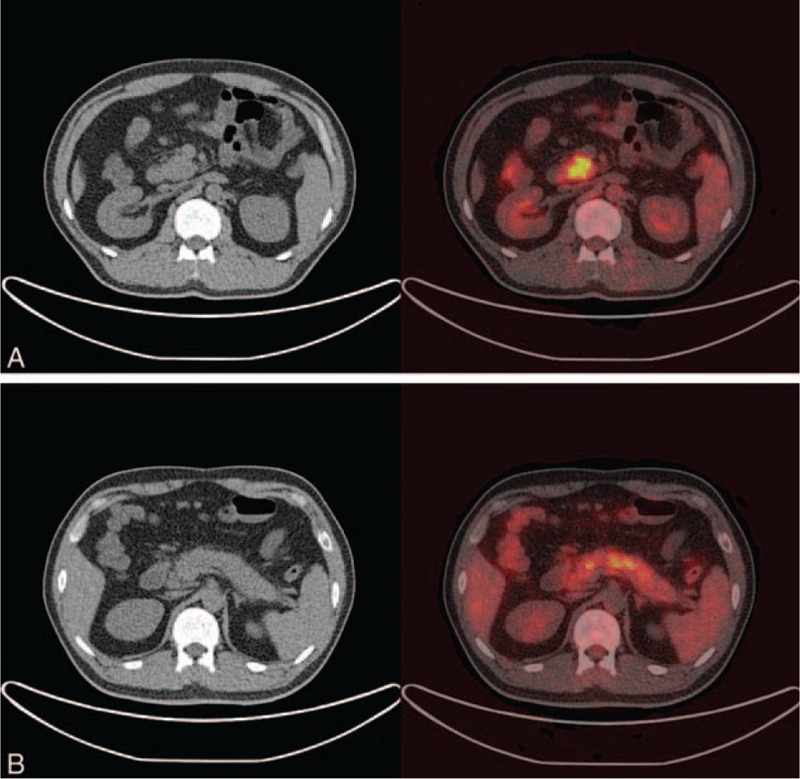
Positron emission tomography/computed tomography body imaging demonstrates (A) a swollen uncinate process of the pancreas with increased uptake, which is suspicious for malignancy, and (B) diffusely increased uptake in the body of pancreas, which is suspicious for secondary inflammation.
